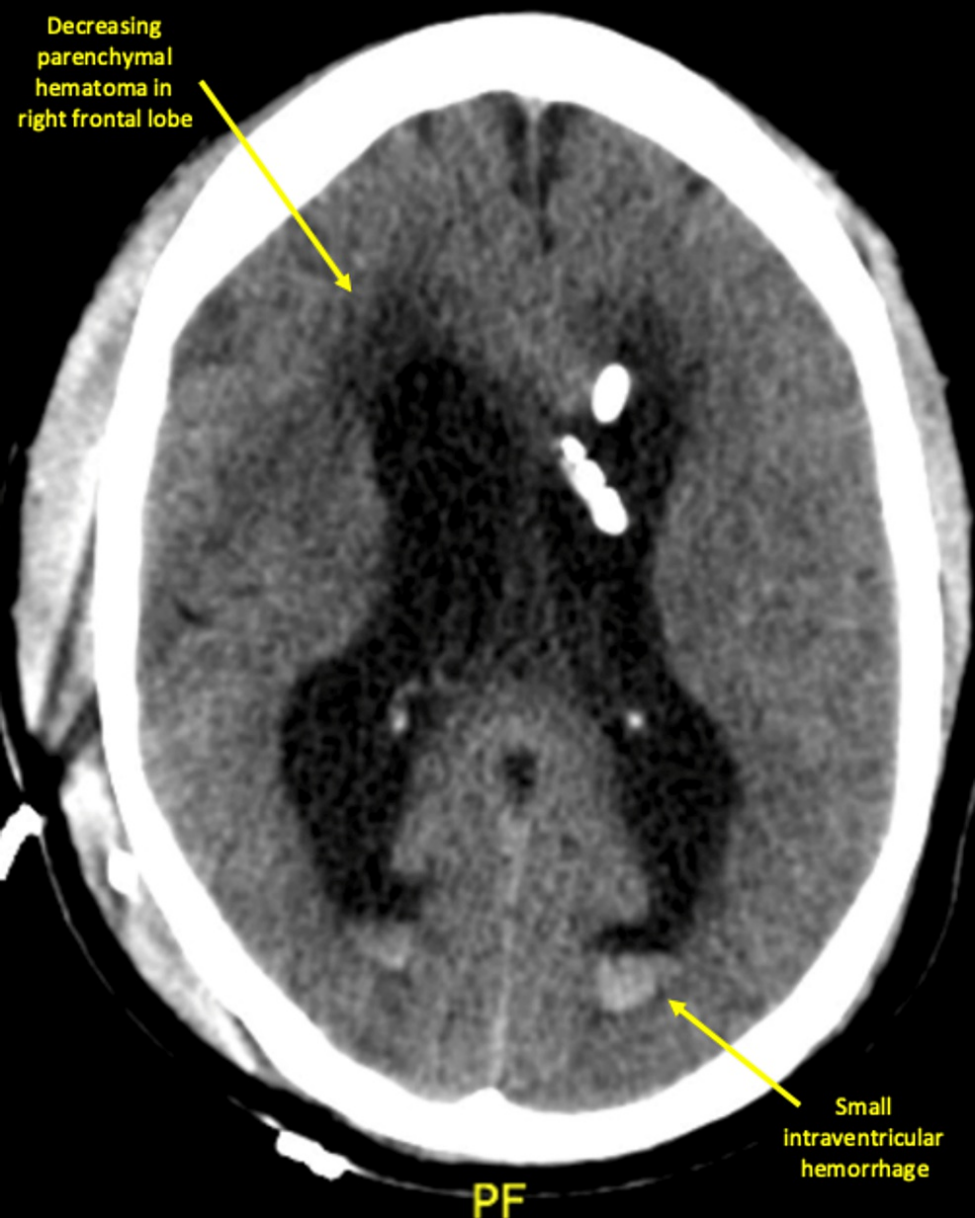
CT head without contrast on day 3 of the first readmission showed a decreasing parenchymal hematoma in the right frontal lobe adjacent to the shunt catheter but only a minimal decrease in size in the lateral and third ventricles. There was also a small amount of intraventricular hemorrhage and a small amount of pneumocephalus. “PF” represents the posterior fossa of the brain.
